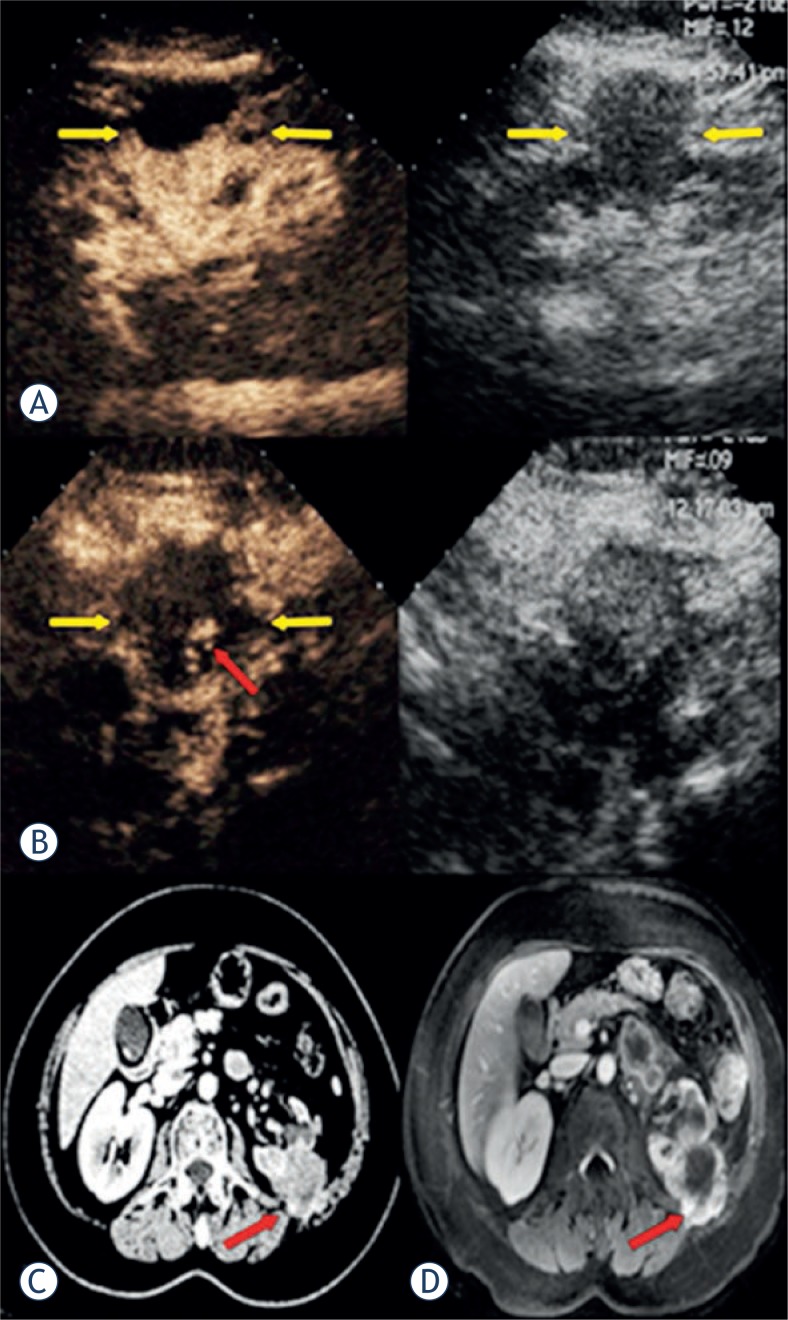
Pre-ablation contrast-enhanced US showed a 4.7 cm × 3.9 cm exophyticly renal papillary cell carcinoma heterogeneous hyper-enhancement with no-enhancement zone in a 56-year-old woman (A, arrow), and 1 month after ablation contrast-enhancement US showed an hyper-enhancement area in cortical phase which was diagnosed as abnormal perfusion (B, red arrow), while the corresponding period CT and MRI showed the hyper-enhancement in article phase and diagnosed as a recurrence tumour (C, D, red arrow). Then the patient received biopsy and another ablation and verified the recurrence.
